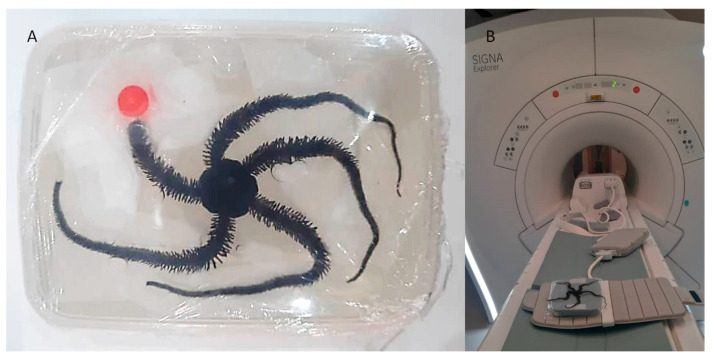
Procedure of MRI imaging of brittle star. (A) The brittle star was placed in a plastic container (10 cm × 15 cm × 5 cm) for MRI procedures. (B) The SIGNA 1.5 T MRI portfolio and Flex coil for arm imaging were used for MRI procedures.
